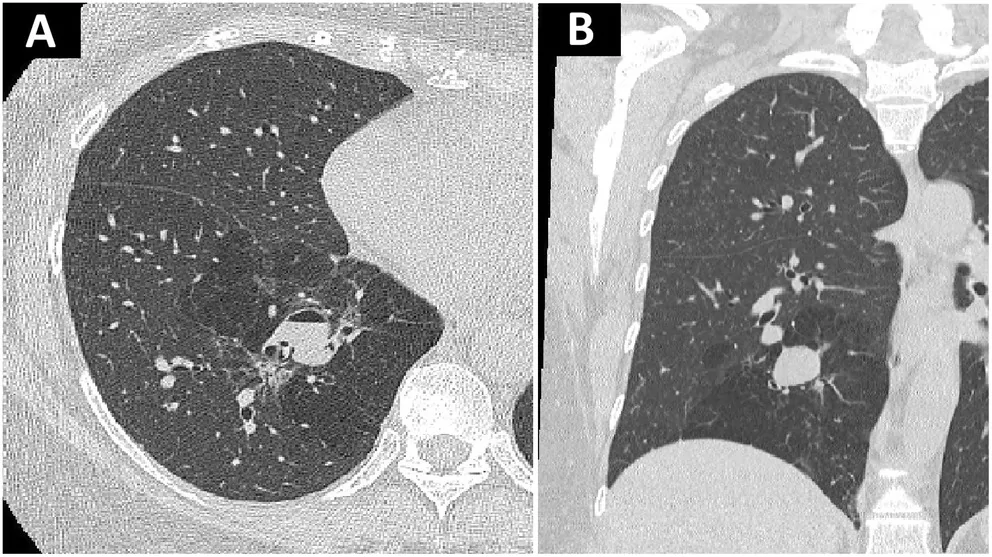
Computed tomography findings at presentation. (A) Axial CT image showing a cavitary lesion with internal fluid retention in Segment 7 (S7) of the right lower lobe, consistent with a bronchocele, accompanied by surrounding ground‐glass opacity suggestive of inflammation. (B) Coronal CT image demonstrating localized hyperinflation confined to S7.
